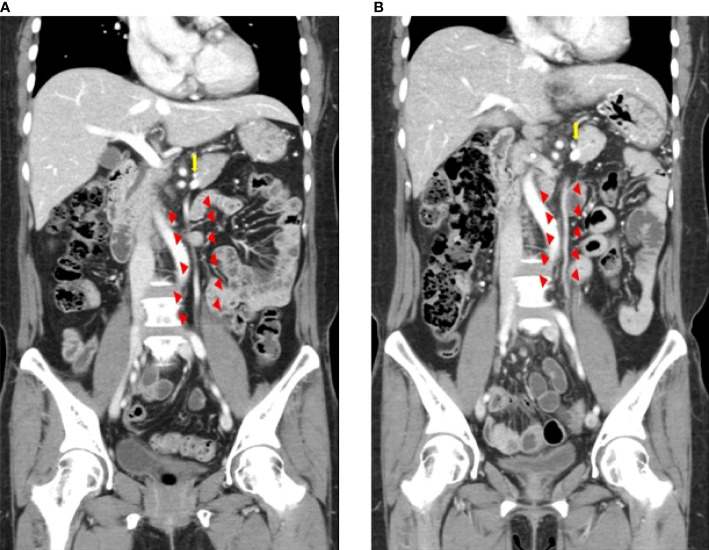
Inferior mesenteric vein preservation confirmed on computed tomography images before (A) and after surgery (B) Triangles indicate the inferior mesenteric vein. The arrow indicates the splenic vein.
